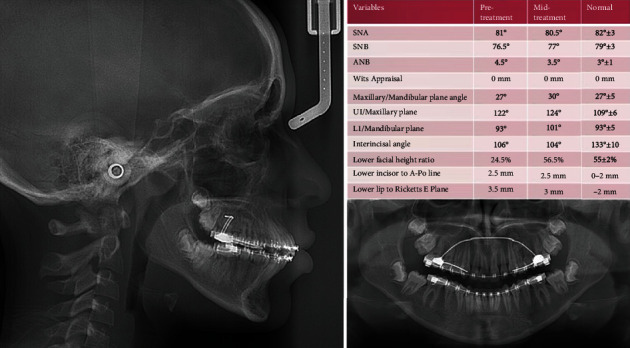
Midtreatment radiographs and analysis.
